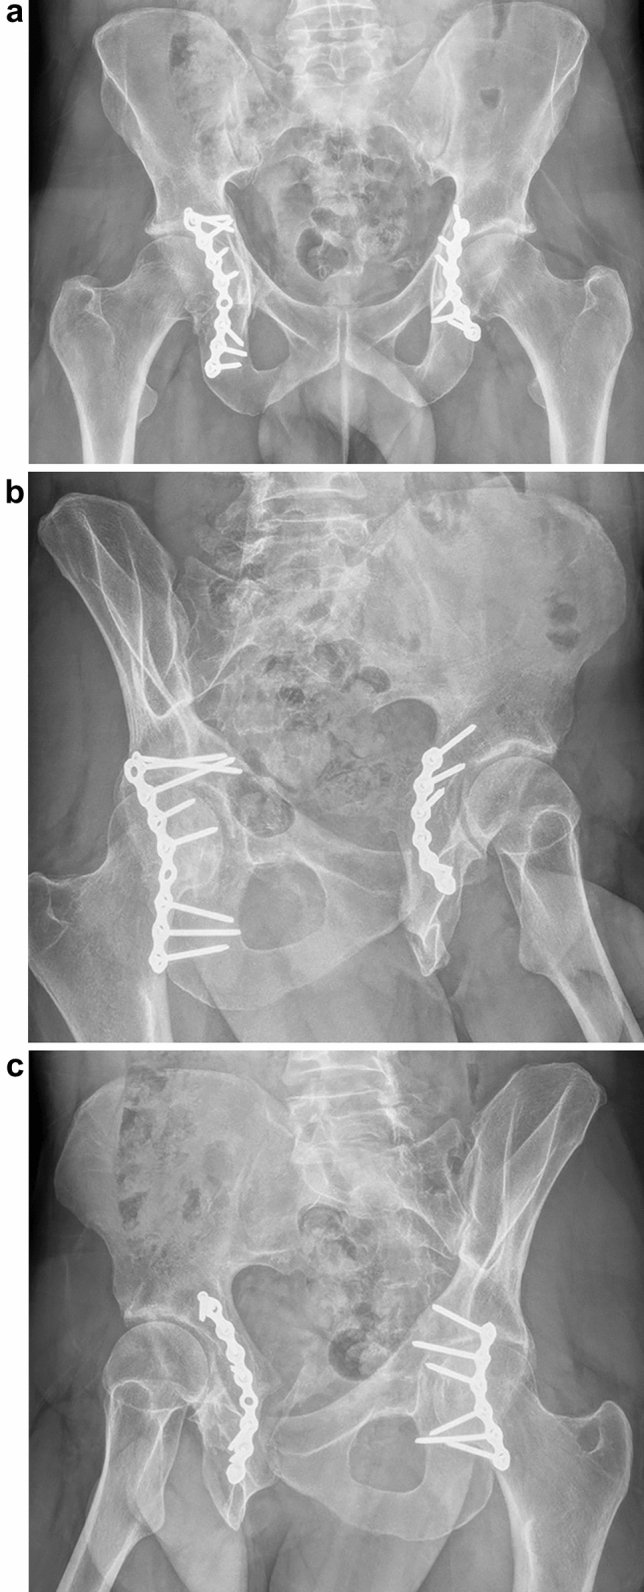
Anteroposterior (a) and Judet view radiographs (b, c) demonstrating osteosynthesis, preservation of hip joint space and united bilateral transverse acetabular fractures at follow-up
